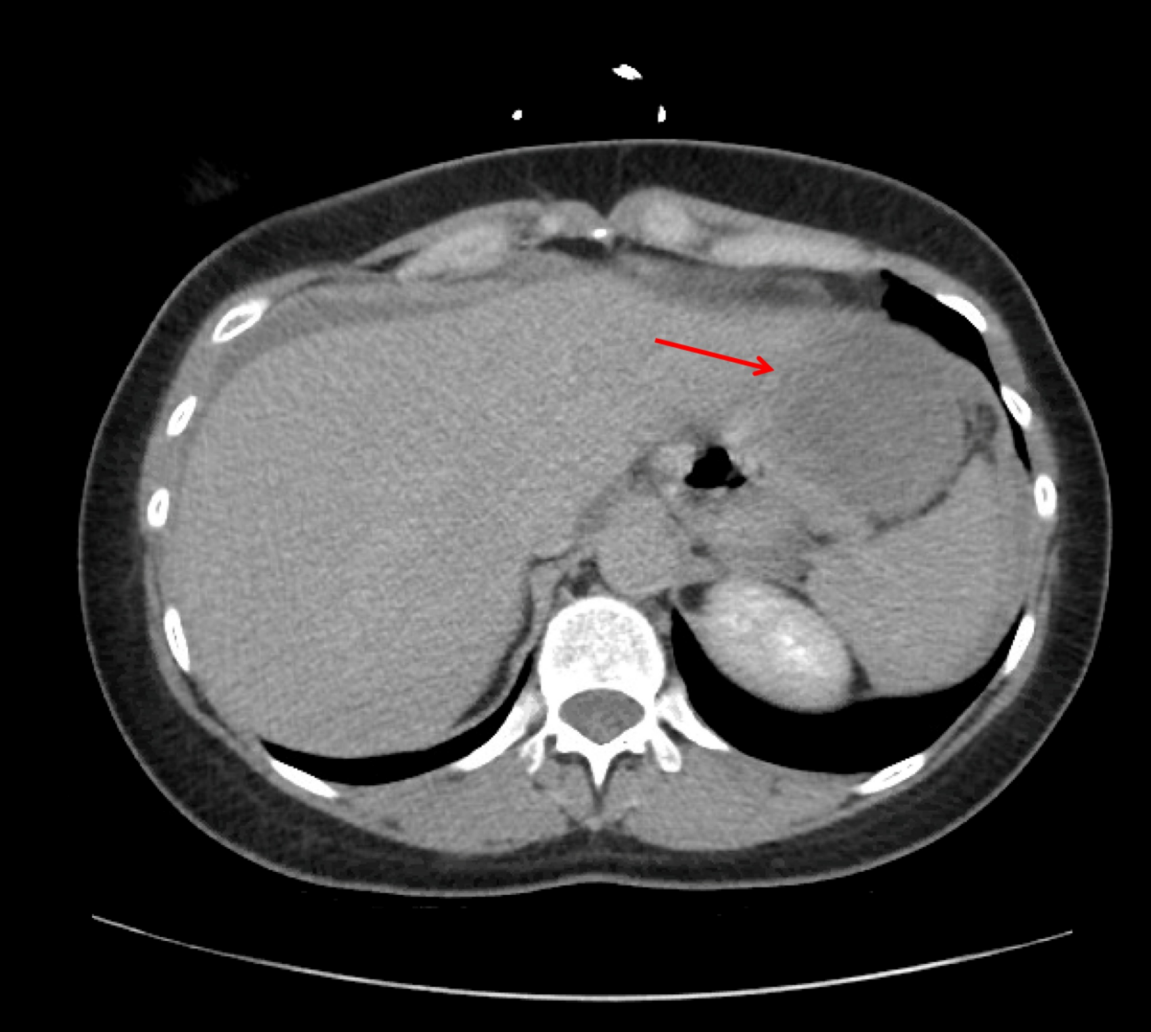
Contrast-enhanced CT abdomen (axial section) showing an irregular heterogeneous mass arising from the anterior wall of the stomach.
